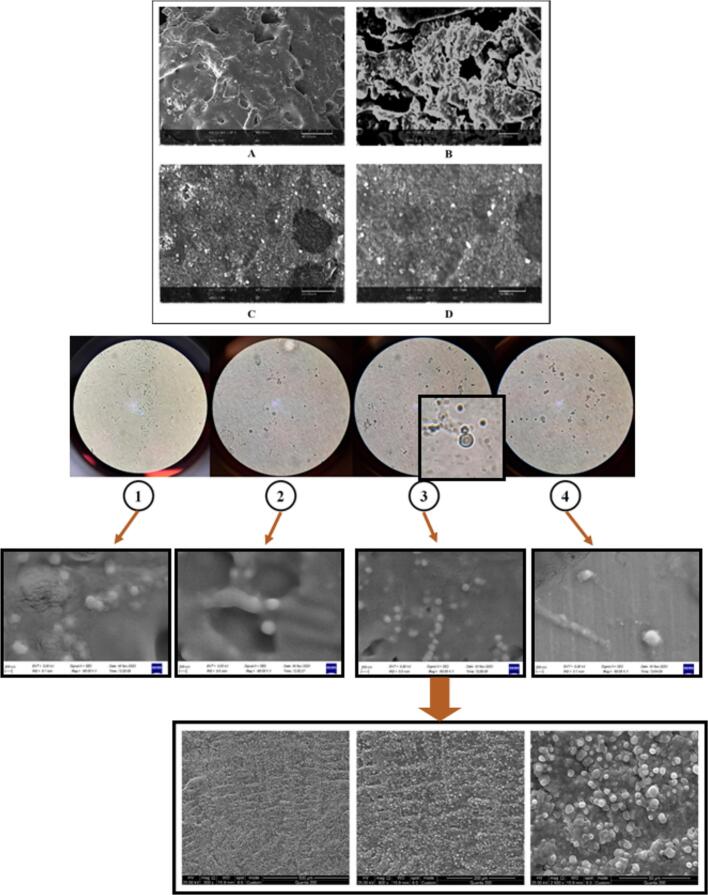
Surface structure images of hydrolyzed protein (A and B correspond to hydrolyzed protein without pretreatment and C and D correspond to hydrolyzed protein with ultrasound pretreatment) and nanoliposomes loaded with hydrolyzed protein of flaxseed (treatments L1 to L4).
